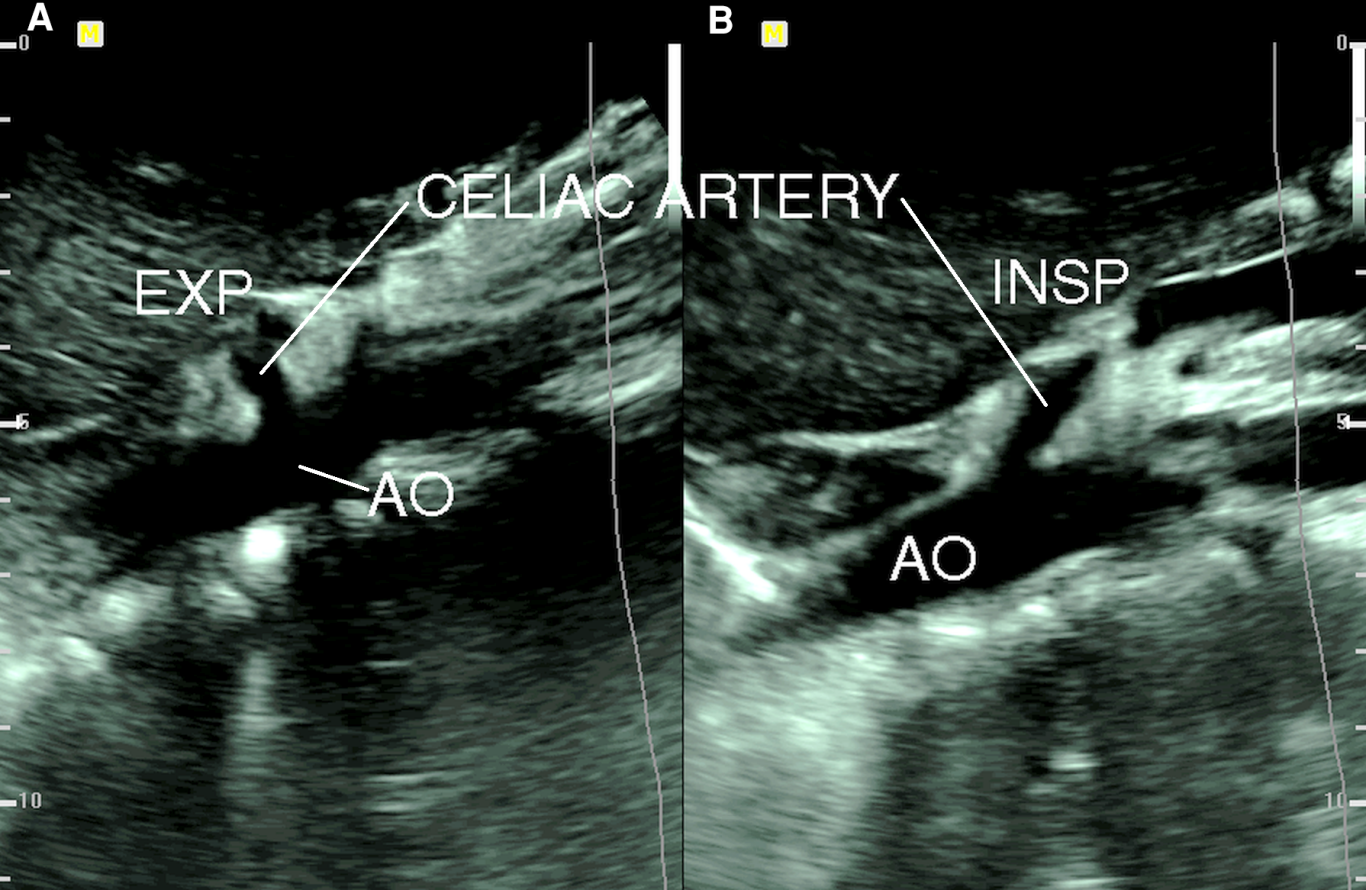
B-mode ultrasound image of celiac artery compression. (A) Celiac artery deflection angle > 50° during expiration (EXP); (B) celiac artery deflection angle < 50° during inspiration (INSP). AO, aorta [from: Youssef (18). Reprint with permission from Dr Youssef].
